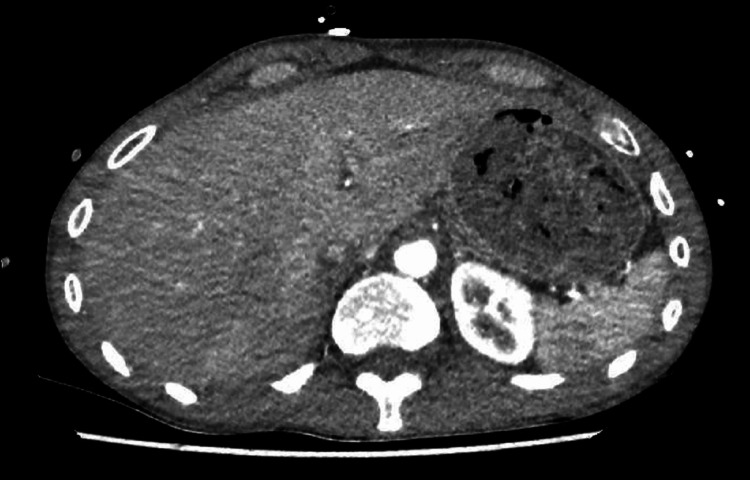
CT of the chest, abdomen, and pelvis No evidence of occult malignancy was seen.
